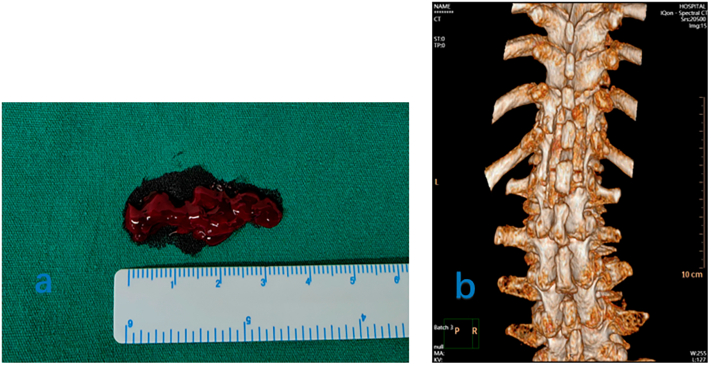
Intraoperative imaging and postoperative CT findings. (a) Clotted blood is removed from the epidural space intraoperatively. (b) CT showing the extent of lamina decompression postoperatively. CT: computed tomography.
